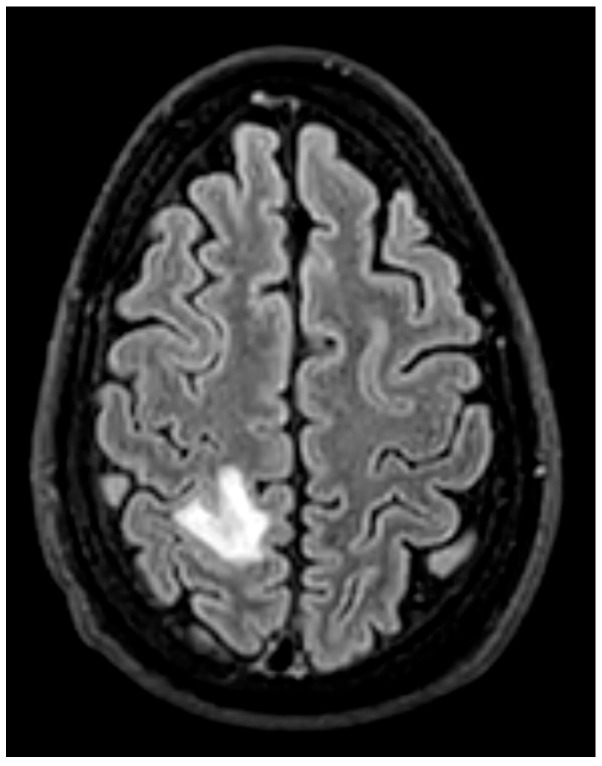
This patient had native aortic valve endocarditis. No neurological symptoms reported; brain magnetic resonance imaging was performed before surgery and showed an embolic lesion in the occipital cortex. This is an original, de-identified clinical image from our institution. Patient consent for publication was obtained.
